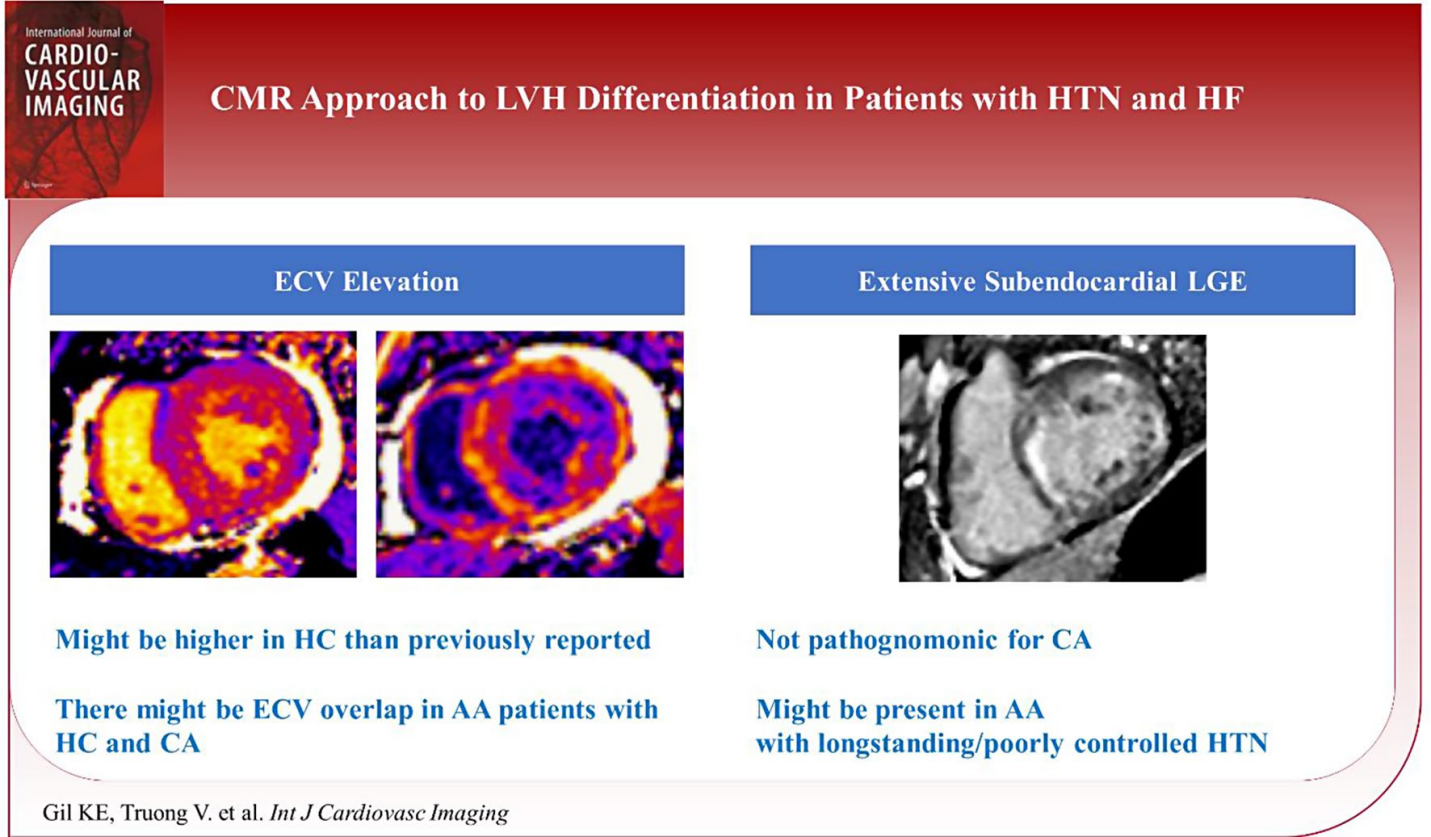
Abbreviations: CMR, cardiovascular magnetic resonance imaging; LVH, left ventricular hypertrophy; HTN, hypertension; HF, heart failure; ECV, extracellular volume fraction; HC, hypertensive heart disease; CA, cardiac amyloidosis; AA, African American; LGE, late gadolinium enhancement
